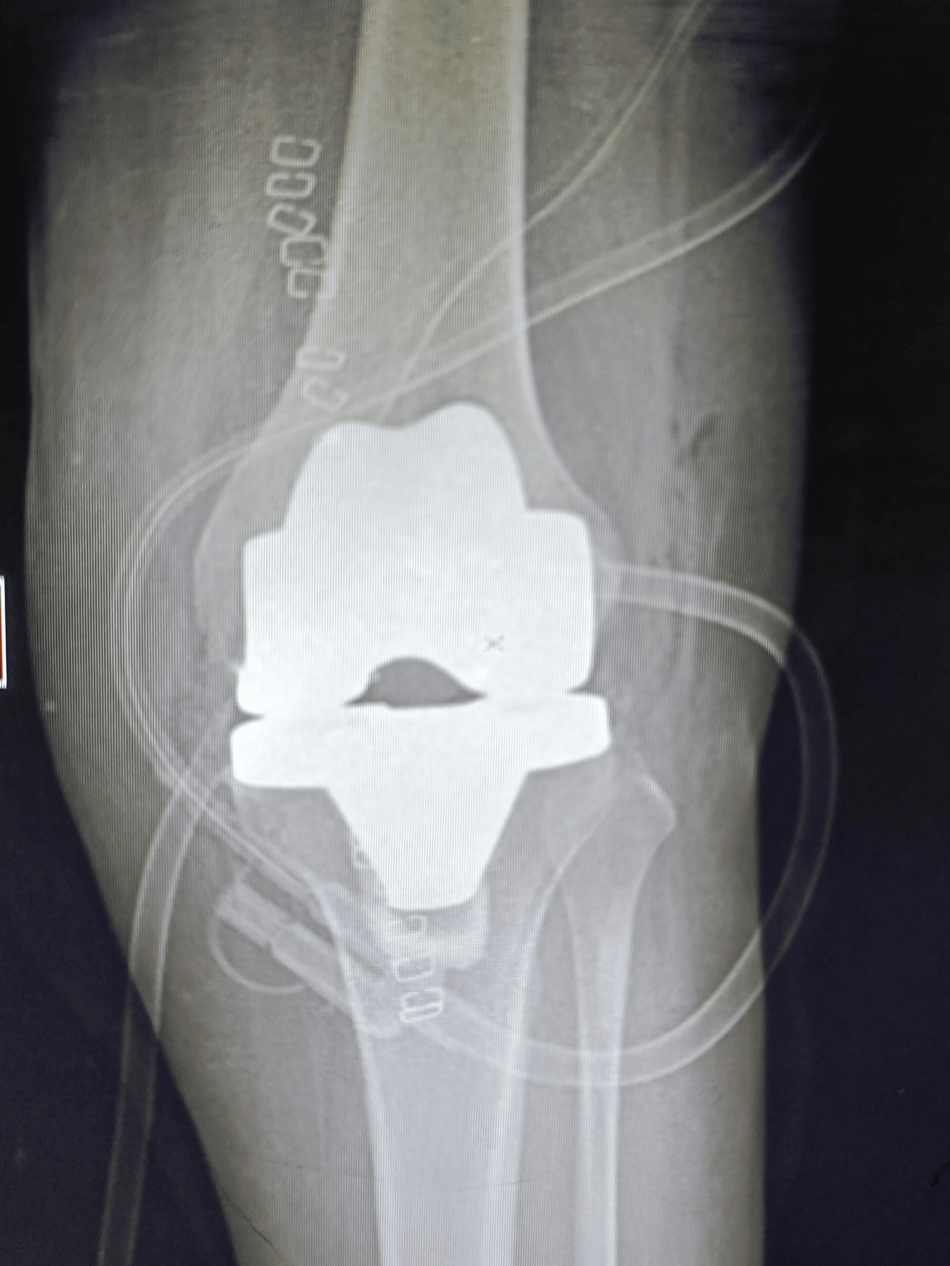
The figure shows the X-ray scan of the patient that was taken after successful surgery
